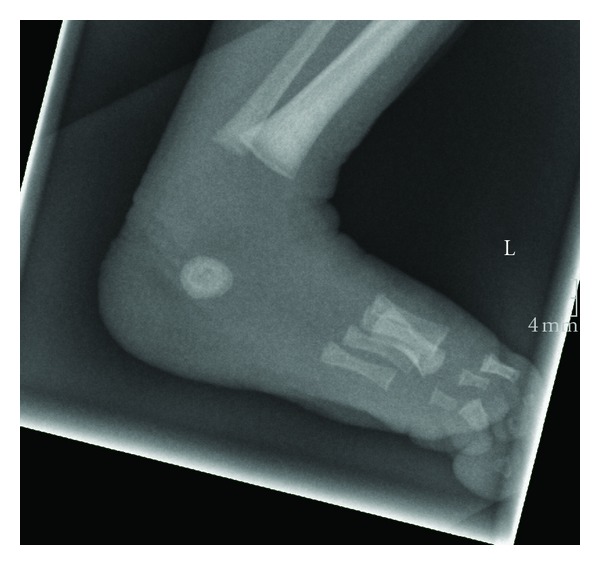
Radiograph of the baby's foot at 20 days of chronological age. Bone age has been classified as 28th–30th gestational week; thus, bone age is significantly retarded (according to the Hernandez/ Erasmie score).
